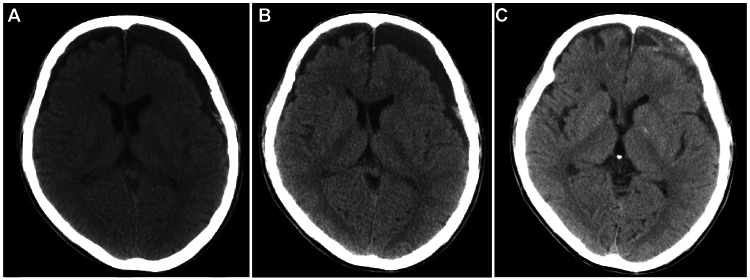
Head CT scan at two weeks (A), three weeks (B), and six weeks (C) after conservative treatment. Air in the cranium disappeared at two weeks, resulting in subdural hygroma, which then developed into a chronic subdural hematoma (C).
